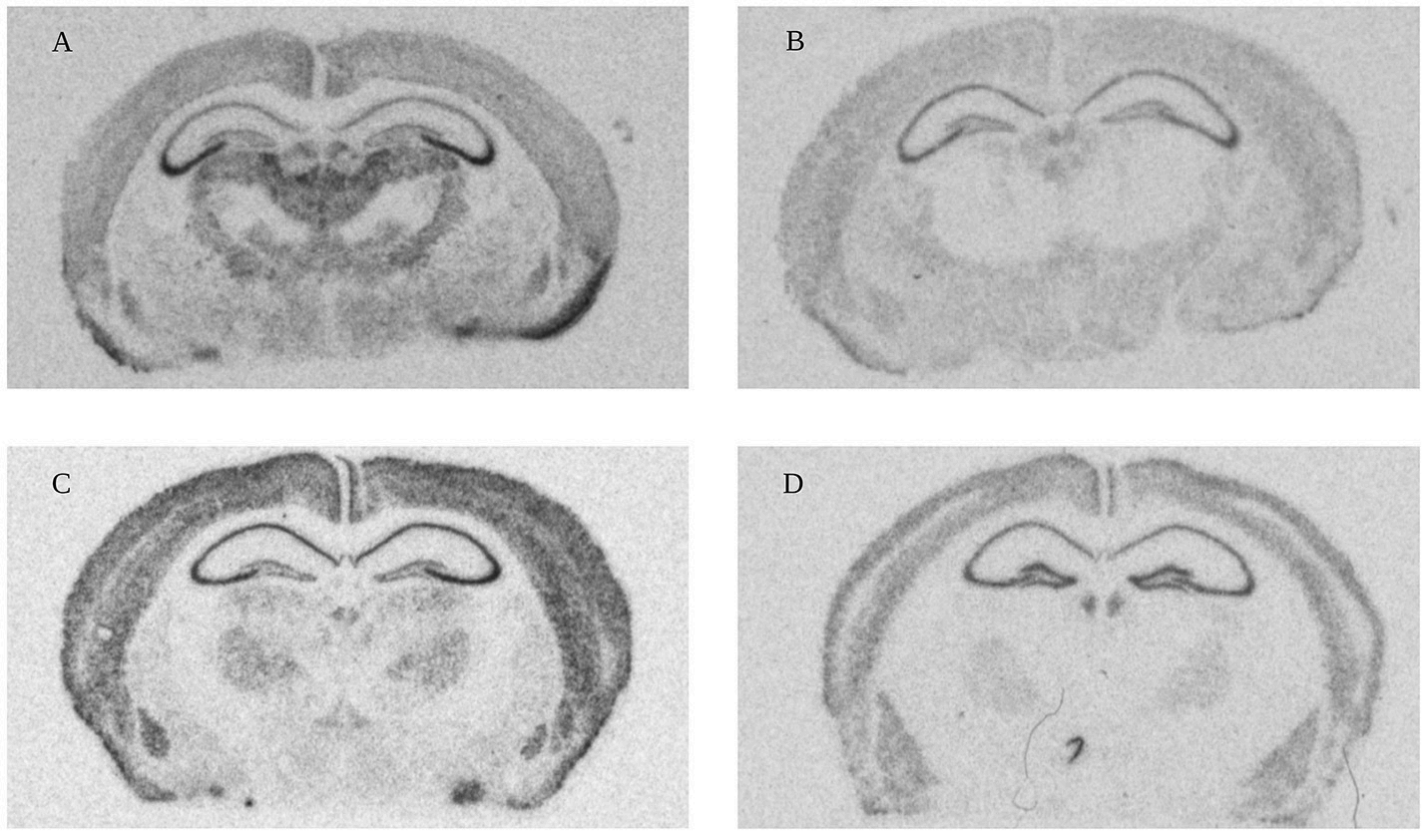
High resolution scans from X-ray film autoradiographs. Representative autoradiograms from *in situ* hybridization performed with ^33^P-labeled oligonucleotides for four different genes (A: Olfactomedin 1 mRNA 1 week; B: Nogo-A mRNA 2 weeks; C: Lingo-1 mRNA 4 weeks and D: NgR1 mRNA 104 weeks). These scans were used for measurements of gene expression in the 18 different regions (Figure 3).
